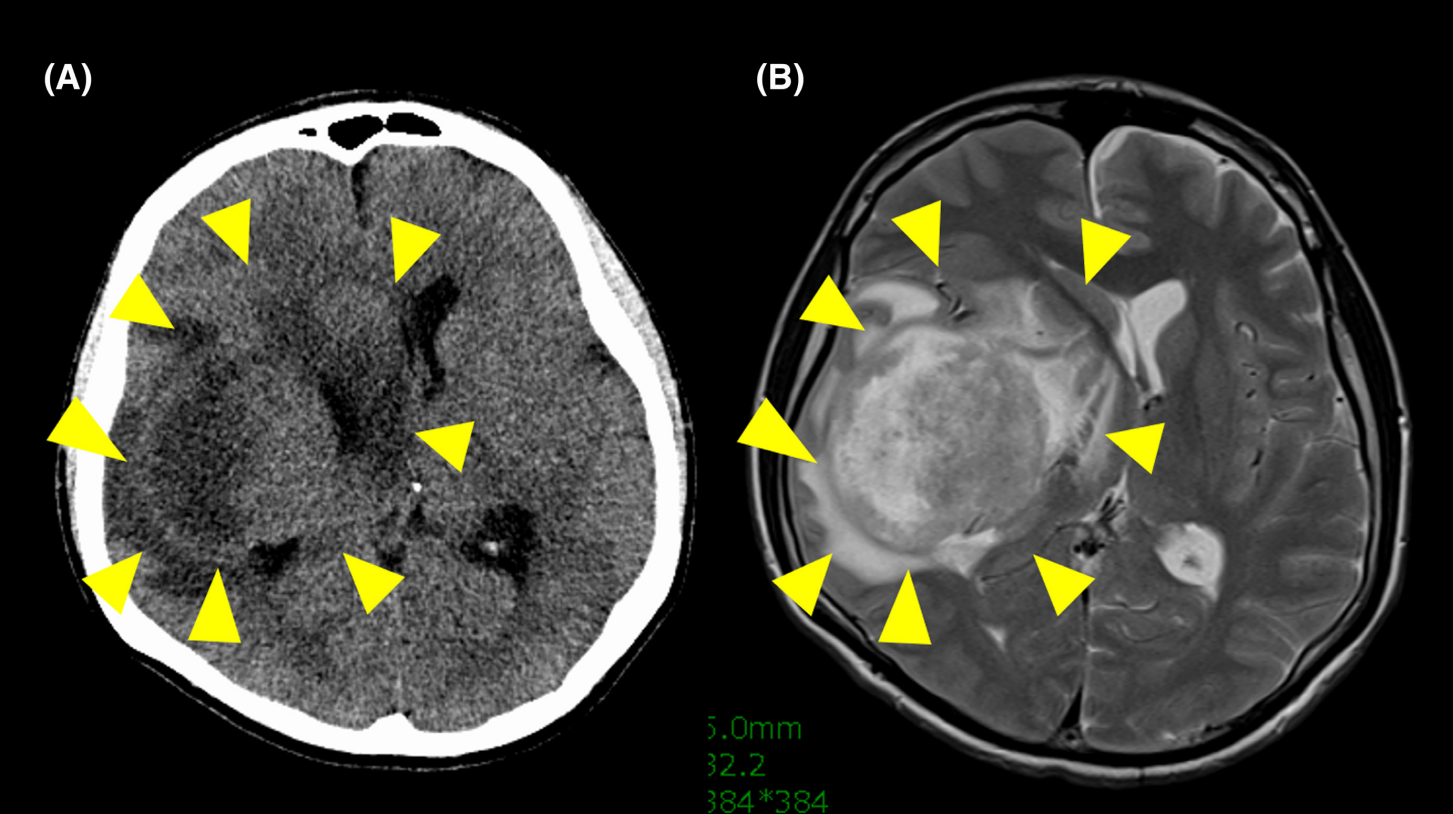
Representative brain computed tomography (A) and T2‐weighted magnetic resonance imaging (B) of brain metastasis of sarcoma (malignant peripheral nerve sheath tumor). A brain tumor in the right cerebral hemisphere (arrow) and a midline shift can be seen.
